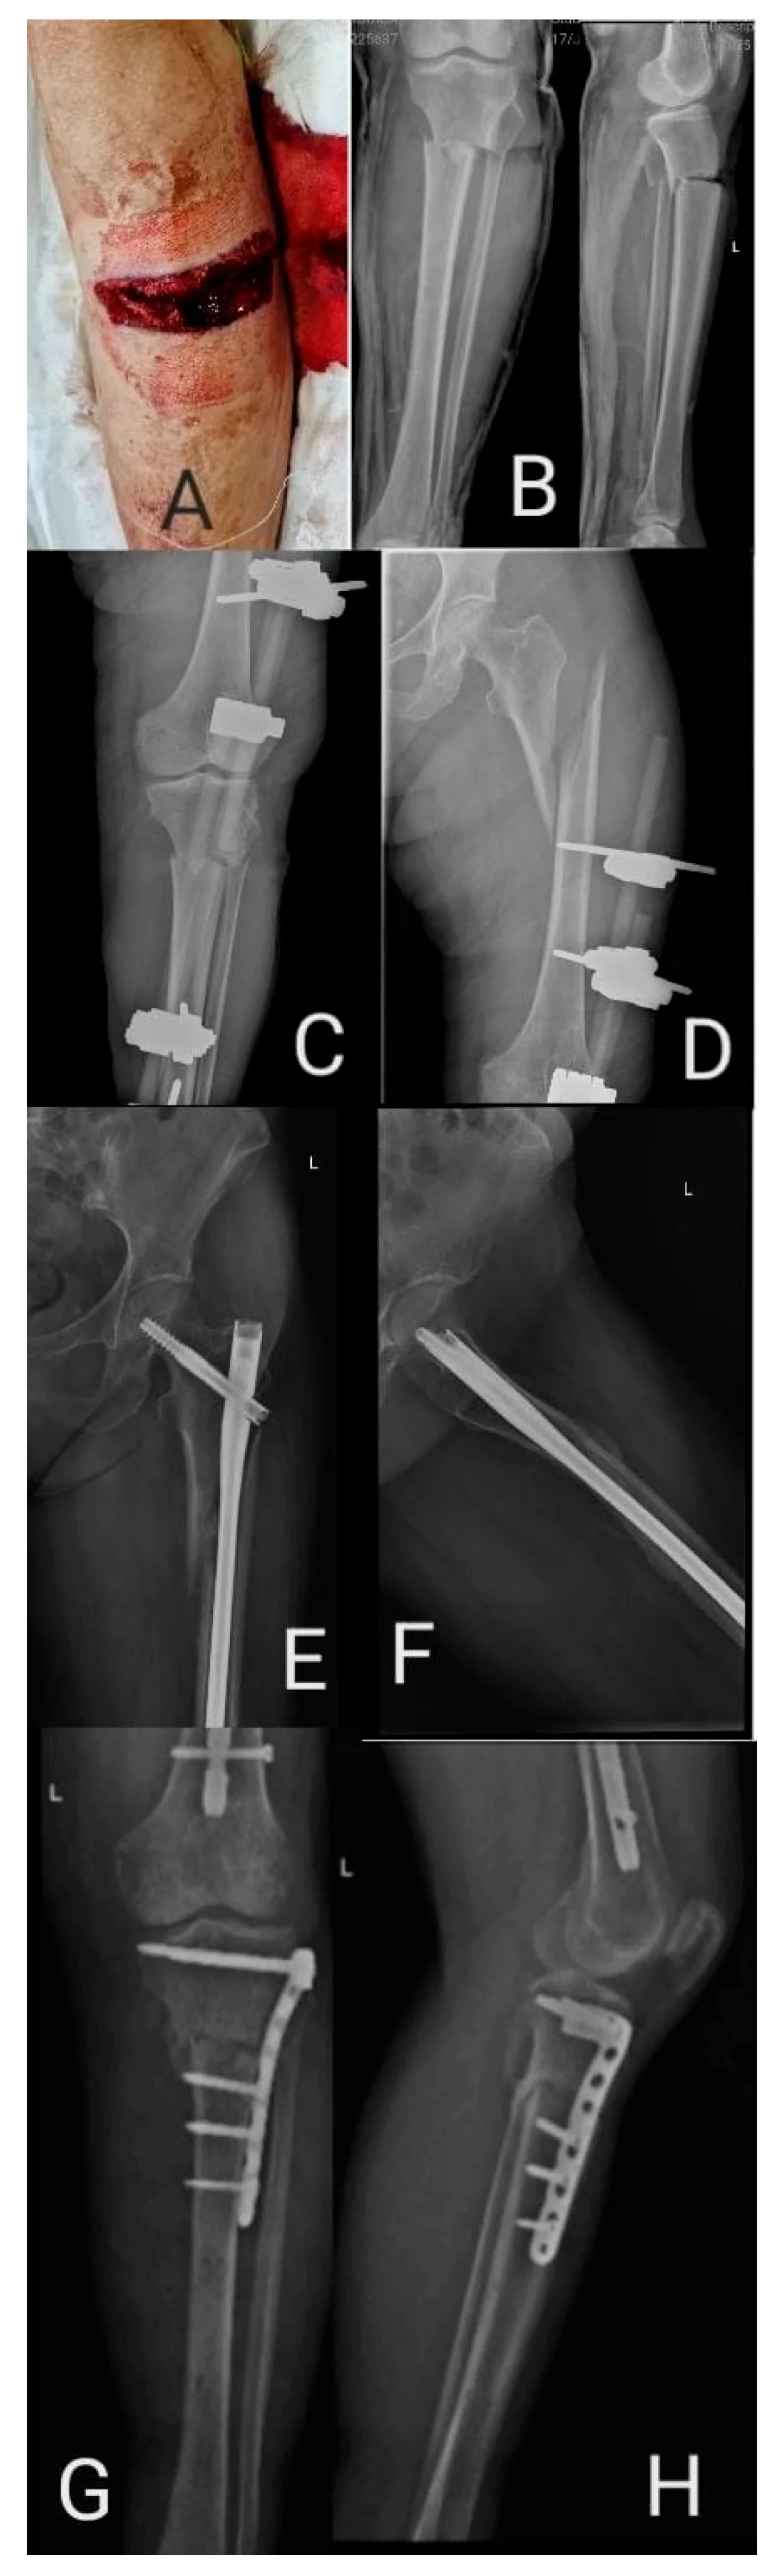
Staged management of an open proximal tibial fracture complicated by alcohol withdrawal-related in-hospital trauma. (A) Clinical appearance of the contaminated open wound associated with the Gustilo–Anderson type IIIA proximal tibial fracture. (B) Initial anteroposterior and lateral radiographs of the proximal tibial fracture. (C) Temporary stabilisation using a knee-spanning external fixator. (D) Subtrochanteric femoral fracture sustained during an in-hospital fall, with the external fixator remaining in situ. (E,F) Anteroposterior and lateral radiographs after closed reduction and fixation of the subtrochanteric fracture with a long Gamma nail. (G,H) Anteroposterior and lateral radiographs after definitive fixation of the proximal tibial fracture with an angular-stable plate.
